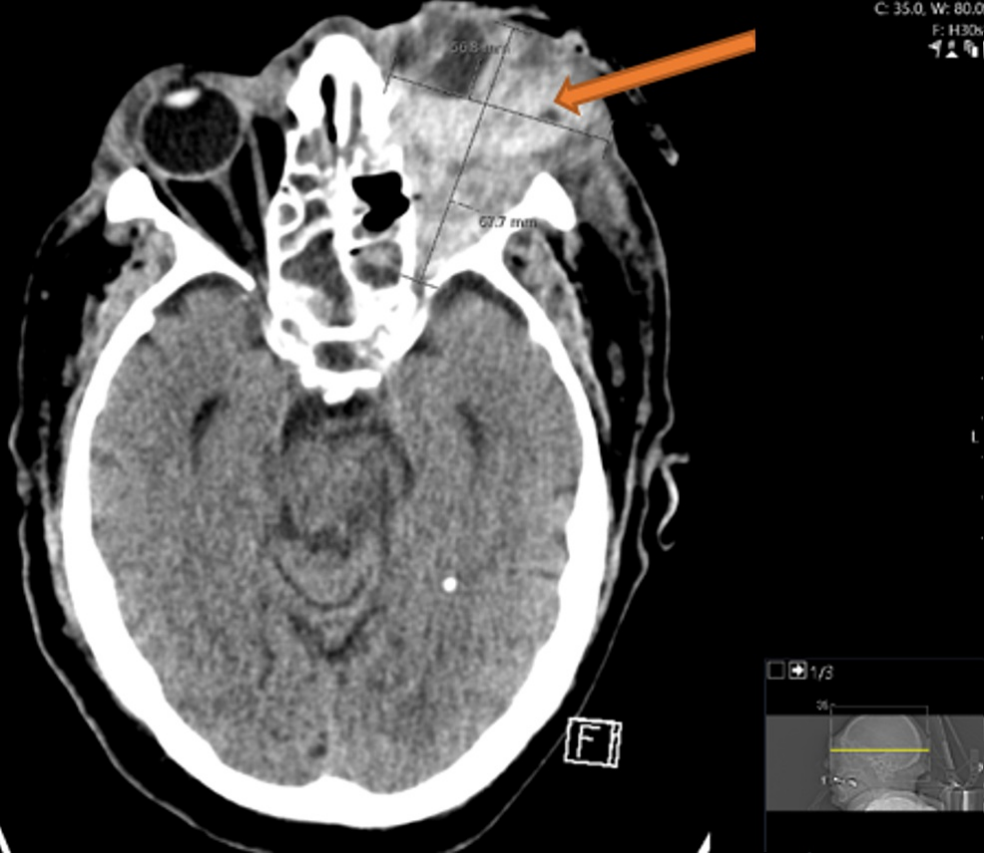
CT of the orbit sella with contrast. Impression: Status post left-sided enucleation with interval development of a large intraorbital hematoma (orange arrow), measuring approximately 67 x 57 mm in maximal anteroposterior and transverse diameter.
